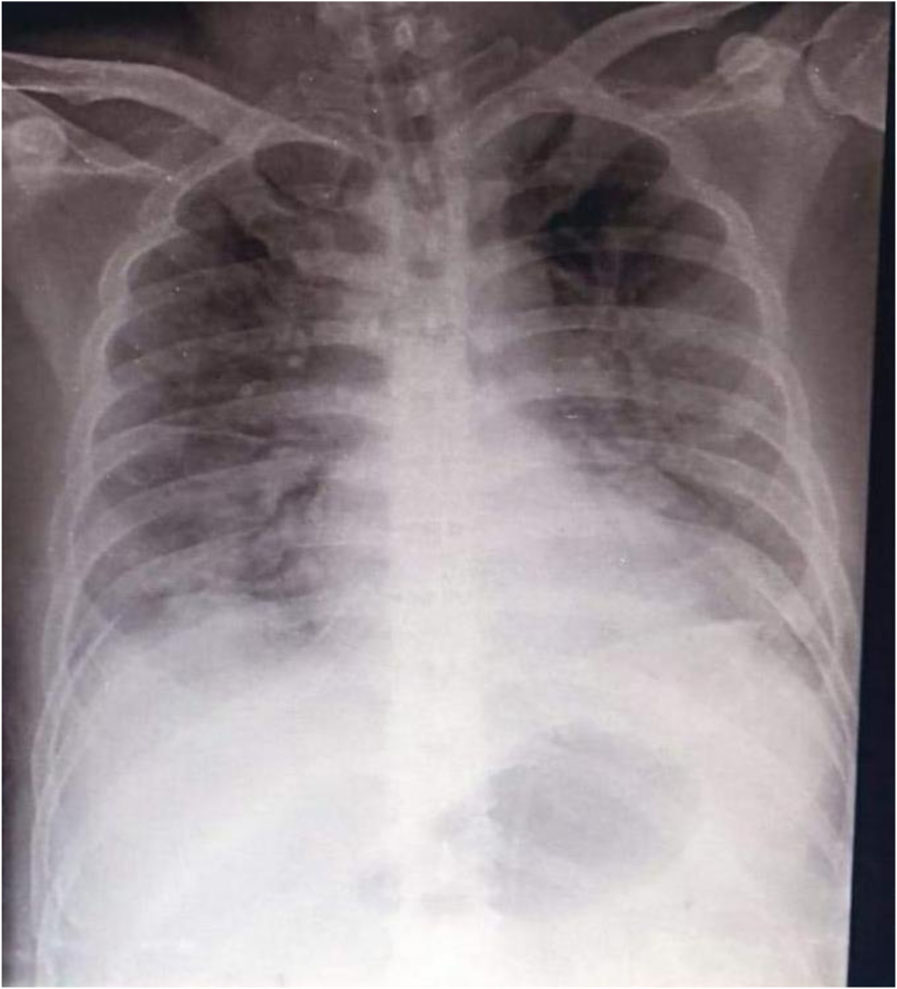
Posteroanterior chest radiograph of patient 2, 30 January 2020 (illness day 13). Endotracheal tube in situ approximately 2 cm above the carina. There is worsening of the previously noted pneumonia
